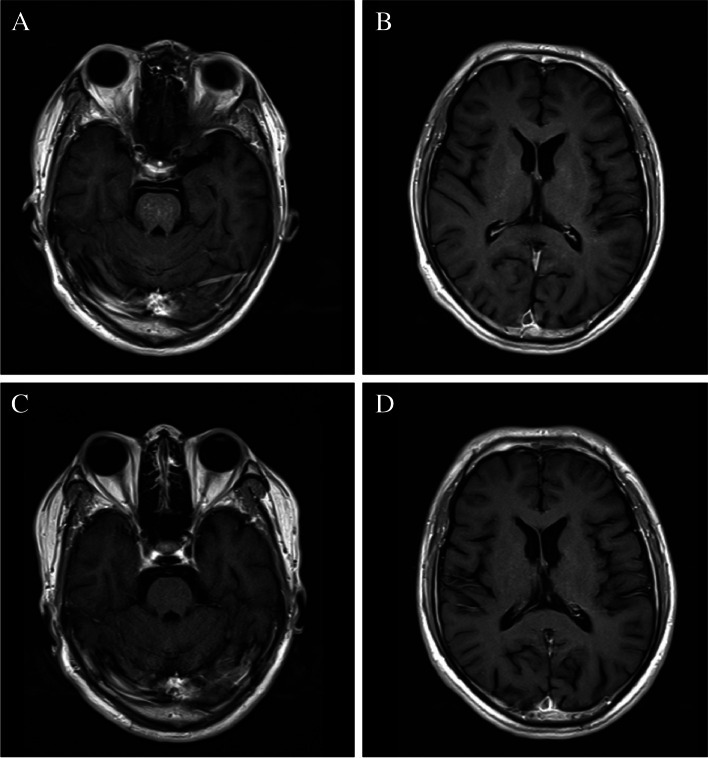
Contrast-enhanced T1-weighted MRI shows that the lesions in the pons, basal ganglia and cerebral hemisphere have been significantly reduced after 1 week of corticosteroid therapy (A and B), and follow-up cranial MRI enhancement shows that the intracranial lesions have almost disappeared after 3 months (C and D)
